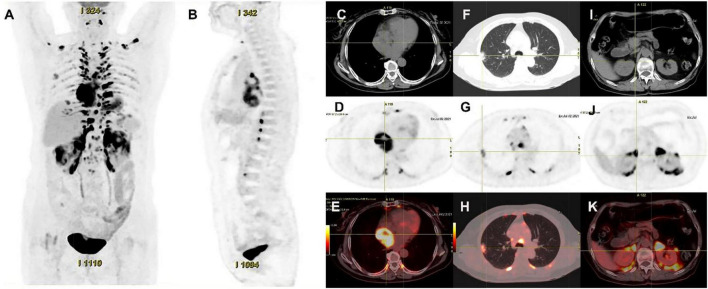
^18^F-FDG PET/CT images of cardiac paraganglioma. (A,B) The whole-body maximum intensity projection showed an intensely hypermetabolic thoracic mass. (C–E) In a cardiac lesion with a high FDG uptake, the SUVmax is about 15.2. (F–H) In the upper lobe of the right lung, a mixed-density nodule with a mild FDG-avid was found near the pleural, with the SUVmax at about 5.8 and a size of about 1.7 cm × 2.3 cm, inflammatory lesions were considered. (I–K) High FDG uptake of brown fat was demonstrated symmetrically along the perirenal and retroperitoneal area with the SUVmax at about 34.4, which was usually caused by the high secretion of catecholamine hormones from paragangliomas.
